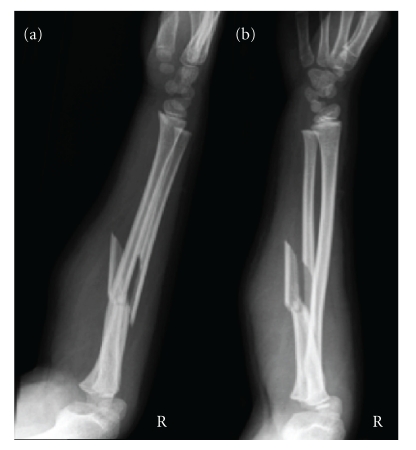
Lateral (a) and oblique (b) radiographs of a right type I Monteggia fracture-dislocation.
